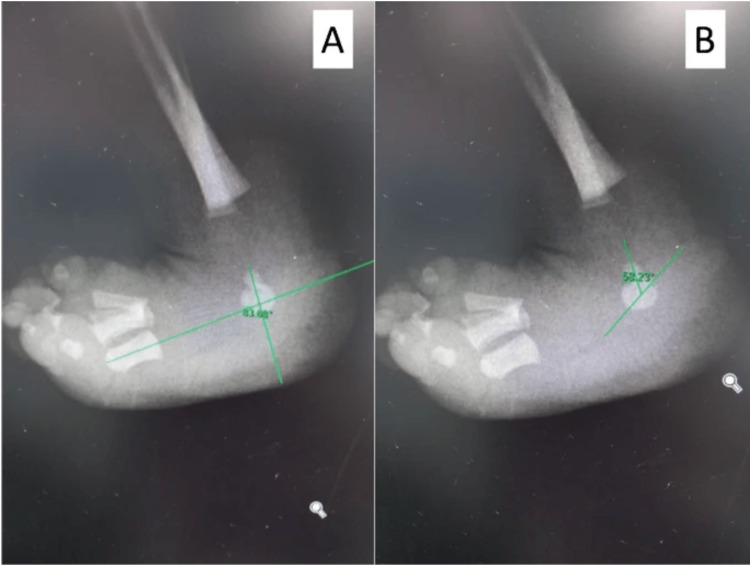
Xray outlining the angles measured A. talo-first metatarsal angle  B. talocalcaneal angle Images courtesy Utrilla-Rodríguez et al. [17] Creative Commons License (CC BY 4.0).
